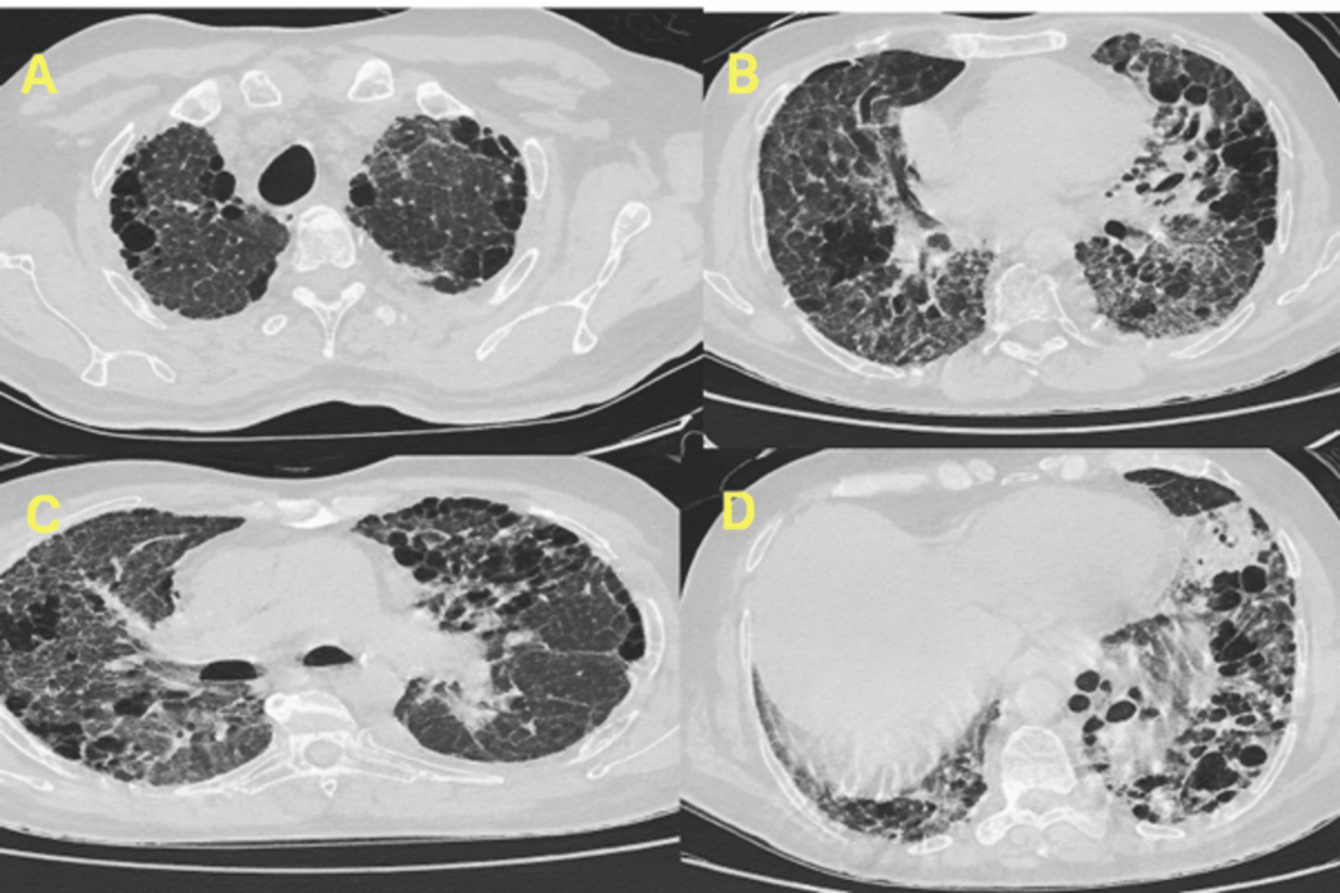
High-resolution CT thorax demonstrating advanced fibrotic interstitial lung disease: Subpleural macrocystic and microcystic honeycombing involving bilateral lung fields, with an apicobasal gradient. Diffuse mild ground-glass opacities with extensive admixed inter- and intralobular septal thickening, predominantly affecting the bilateral lower lobes. Fibrosis with traction bronchiectasis and architectural distortion, most marked in the lower lobes. A. Apical section: Extensive subpleural and peribronchovascular clustered cystic airspaces consistent with honeycombing, predominantly involving the bilateral upper lobes. B. Middle lung zones: Marked architectural distortion, traction bronchiectasis, and reticular opacities with continued honeycombing. C. Lower lung fields: Diffuse reticulation, traction bronchiectasis, and confluent cystic changes with basal predominance. D. Basilar section: Severe fibrotic changes, including extensive subpleural honeycombing with peripheral and lower lobe predominance, consistent with a usual interstitial pneumonia (UIP) pattern. These imaging findings correlate with advanced fibrotic interstitial lung disease, likely definitive UIP pattern.
